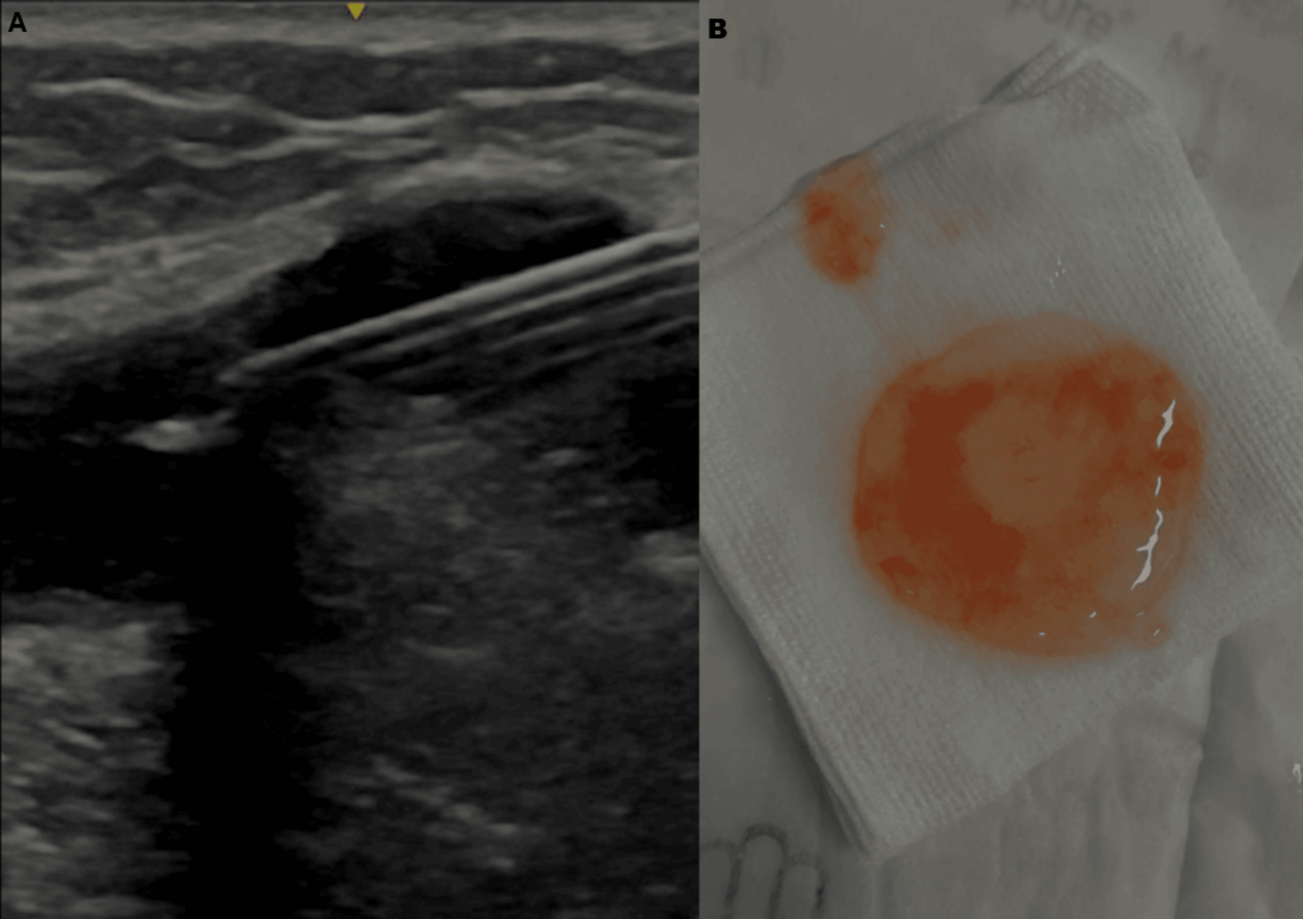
Ultrasound-guided aspiration of a proximal tibiofibular joint ganglion cyst (A) showing needle placement within the lesion, and gross appearance of the aspirated mucinous material with viscous gelatinous content and mild hematic admixture (B).
